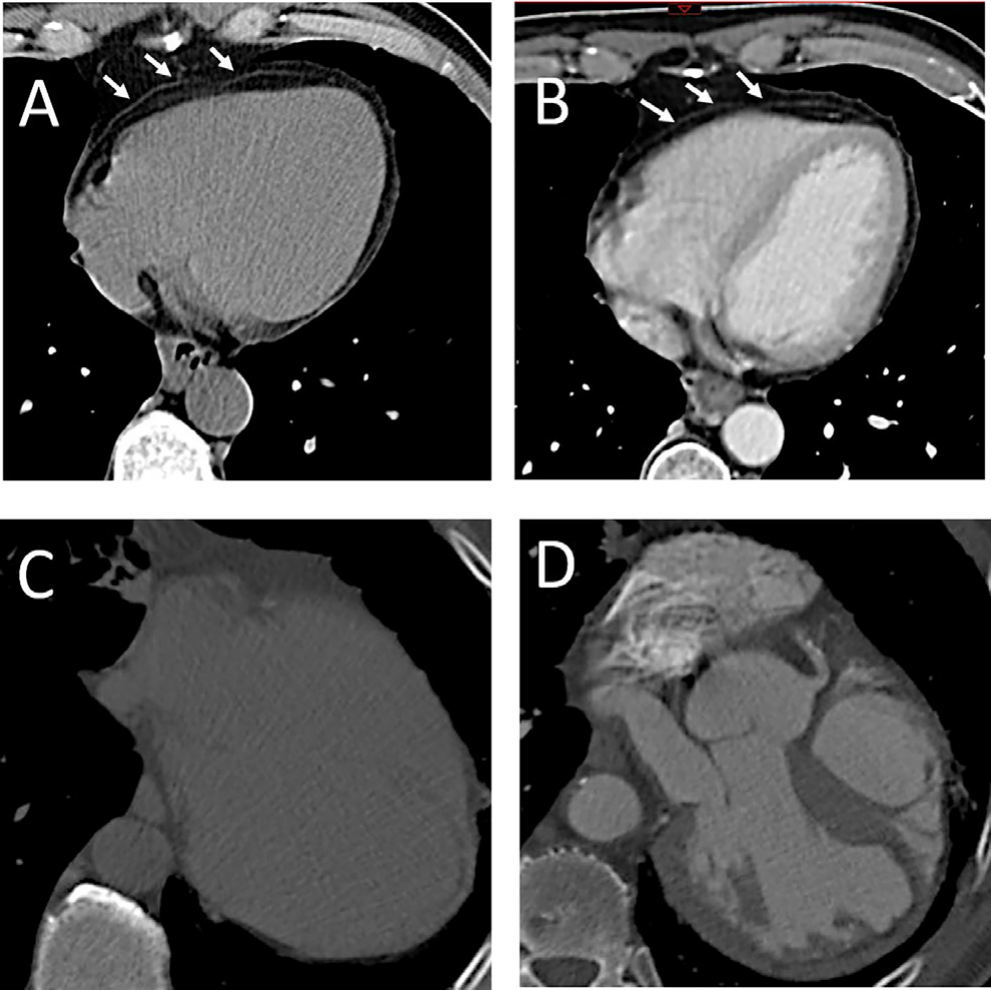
Cardiac computed tomography imaging normal pericardium and congenital absence (A, C) *without contrast agent,* (B, D) *with contrast agent: (A, B) Transaxial section show the pericardium like linear band with a thickness of less than 2 mm (arrow); (C, D) Transaxial section the linear band is not apparent due to the absence of the pericardium.*
